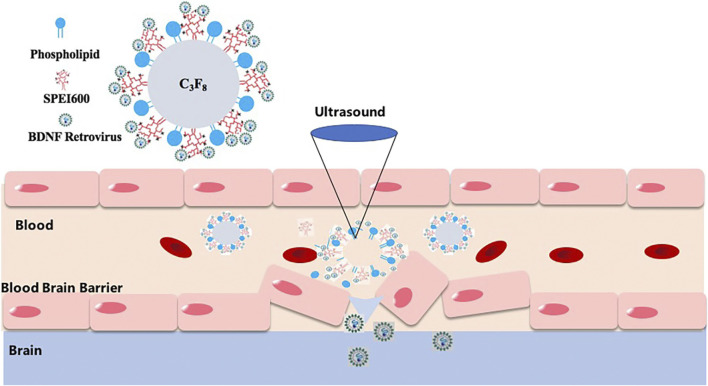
Technology road map of MRI-guided focused ultrasound-induced blood–brain barrier disruption to deliver BDNF retrovirus into the brain. BDNF retrovirus is released to the target cell in the brain after the microbubbles destroying and inducing blood–brain barrier disruption by MRI-guided focused ultrasound at the site of the target brain tissue.
